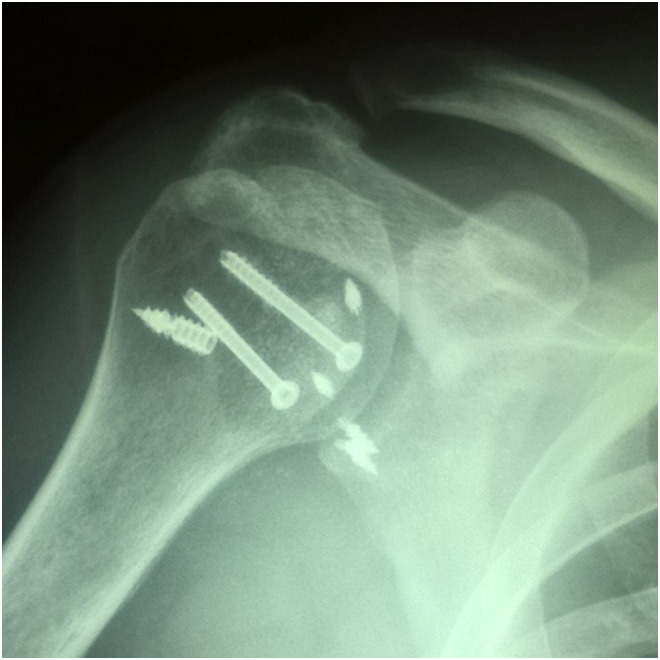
1st patient: Postoperative Right shoulder (AP) radiographs, after the second operation of shoulder arthroscopy and reverse Bankart repair with two metal anchors in posteroinferior glenoid rim.
